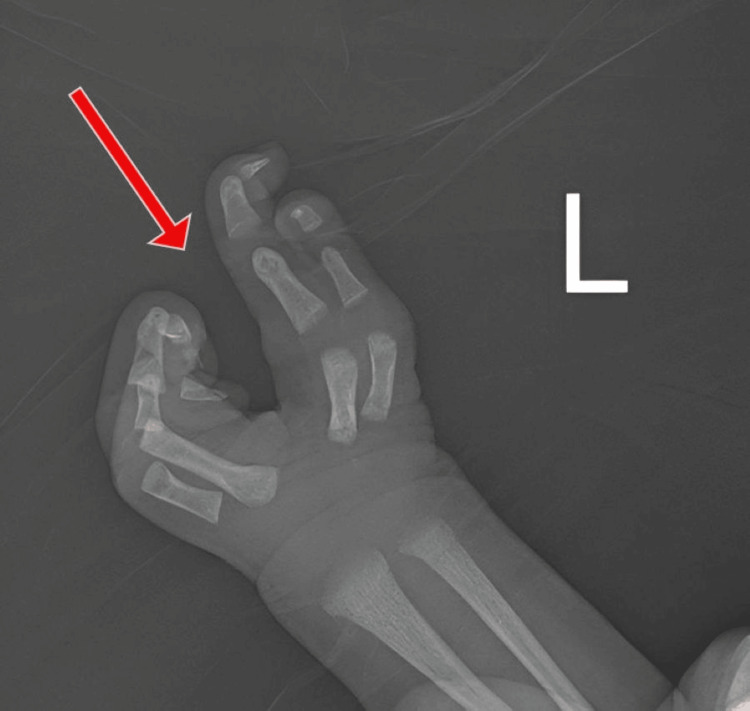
Plain radiograph of the left hand showing the absence of the middle-finger phalanges and metacarpal
